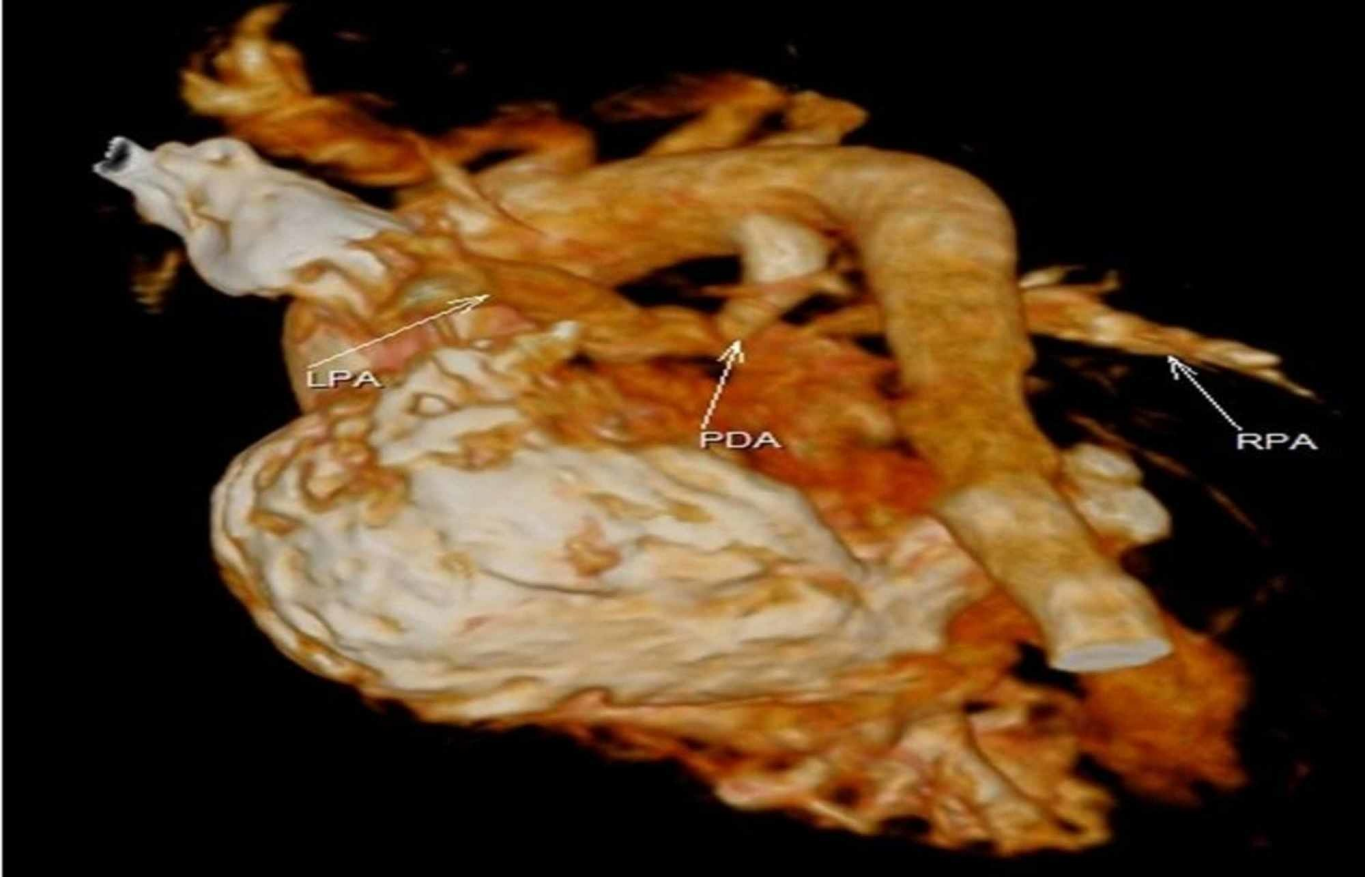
Cardiac volume computed tomography showing anomalous positioning of LPA with PDA and aortic discordance RPA, right pulmonary artery; LPA, left pulmonary artery; PDA, patent ductus arteriosus
